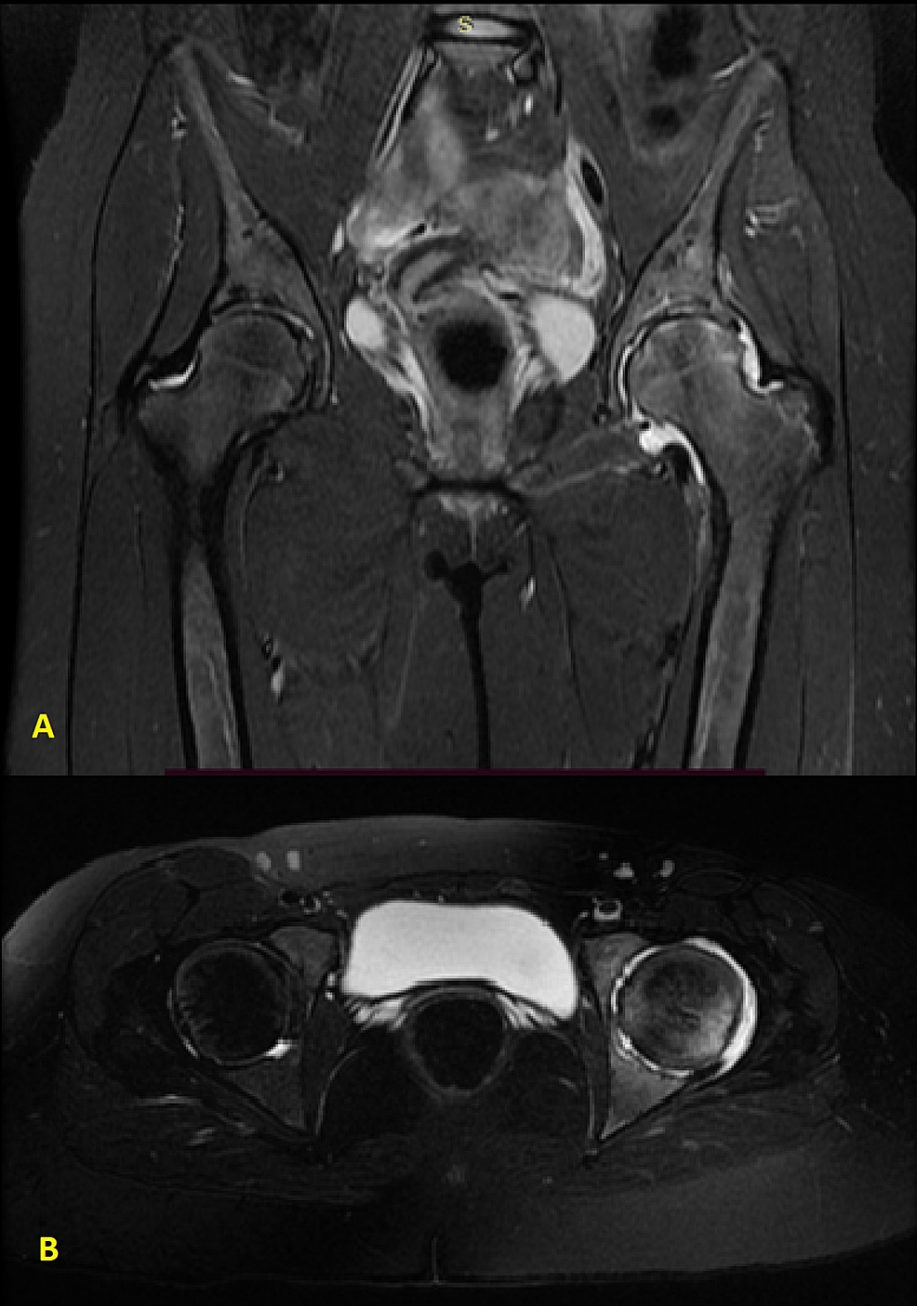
A. Coronal T1-weighted MRI with an abnormal signal of the left epiphysis and synovial thickening. B. Axial T2-weighted MRI showing increased intraarticular fluid and epiphyseal oedema.
